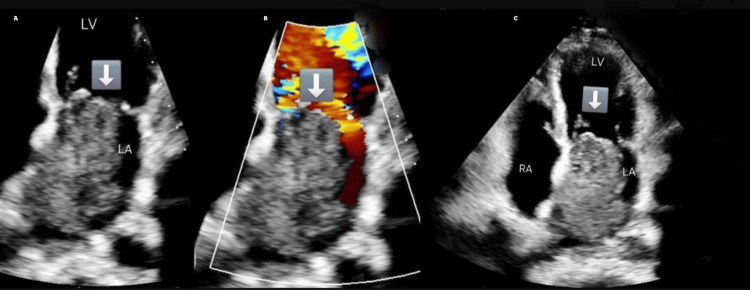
Multimodal imaging of transthoracic echocardiographic findings in left atrial myxoma (A) Two-chamber view showing a large left atrial mass with evidence of left ventricular inflow obstruction with a mean pressure gradient of 15 mmHg. (B) Apical four-chamber view showing a large left atrial mass attached to the interatrial septum. (C) Two-chamber view showing a large mass in the cavity of the left atrium
